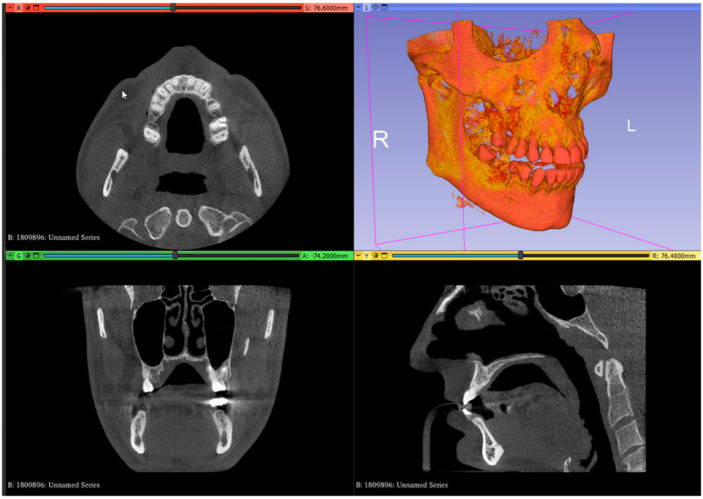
Uploaded CBCT scan in the analysis and scientific visualization software.
